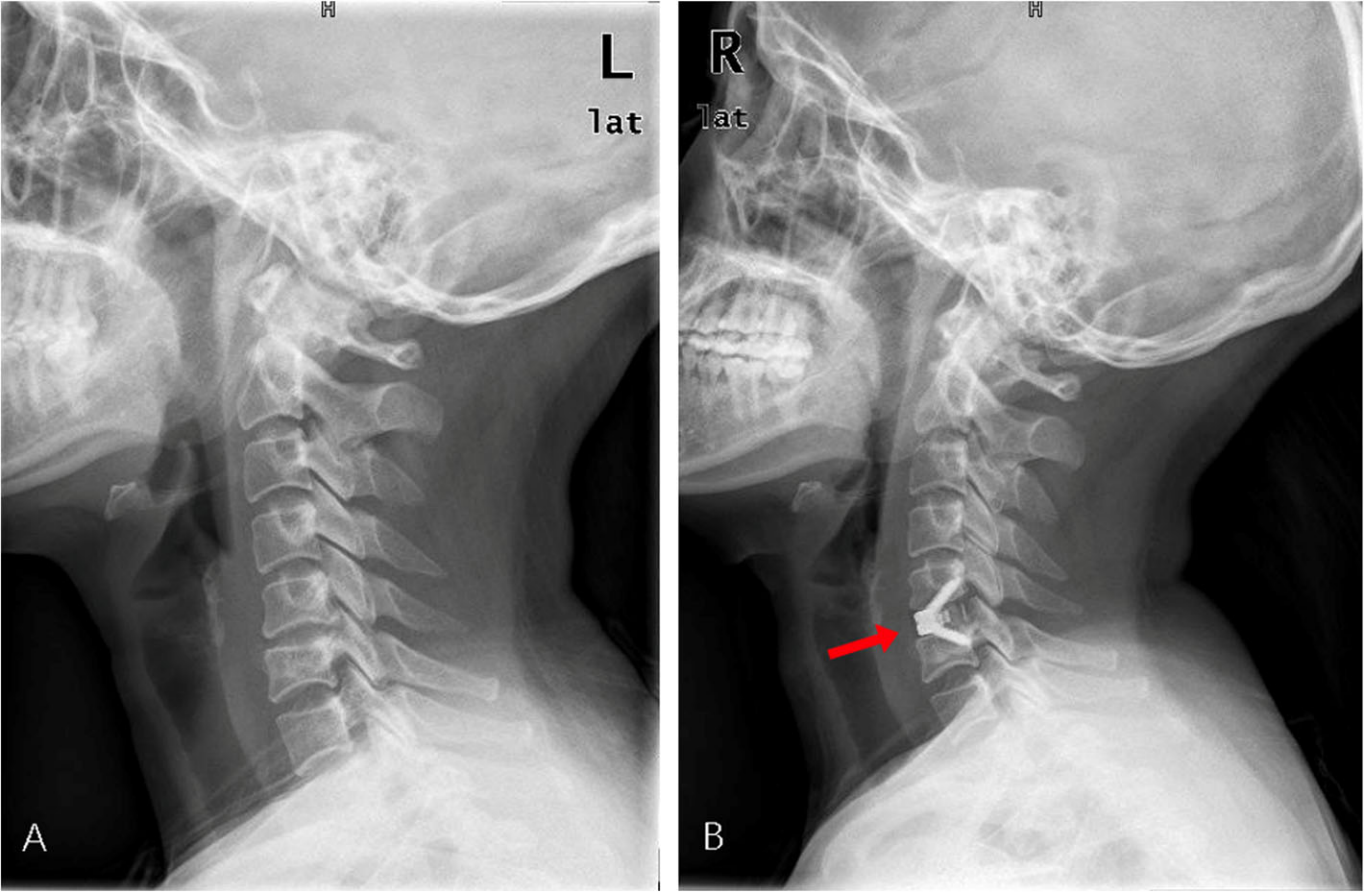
The preoperative (a) and postoperative (b) lateral X-rays of a 40-year-old female patient who was diagnosed with cervical spondylotic myelopathy and underwent C5/6 ACDF with Zero-P. The dO-C_2_A was 8.62°. The Bazaz grading system showed mild postoperative dysphagia. The X-rays showed obvious dC_2–7_A (red arrow)
